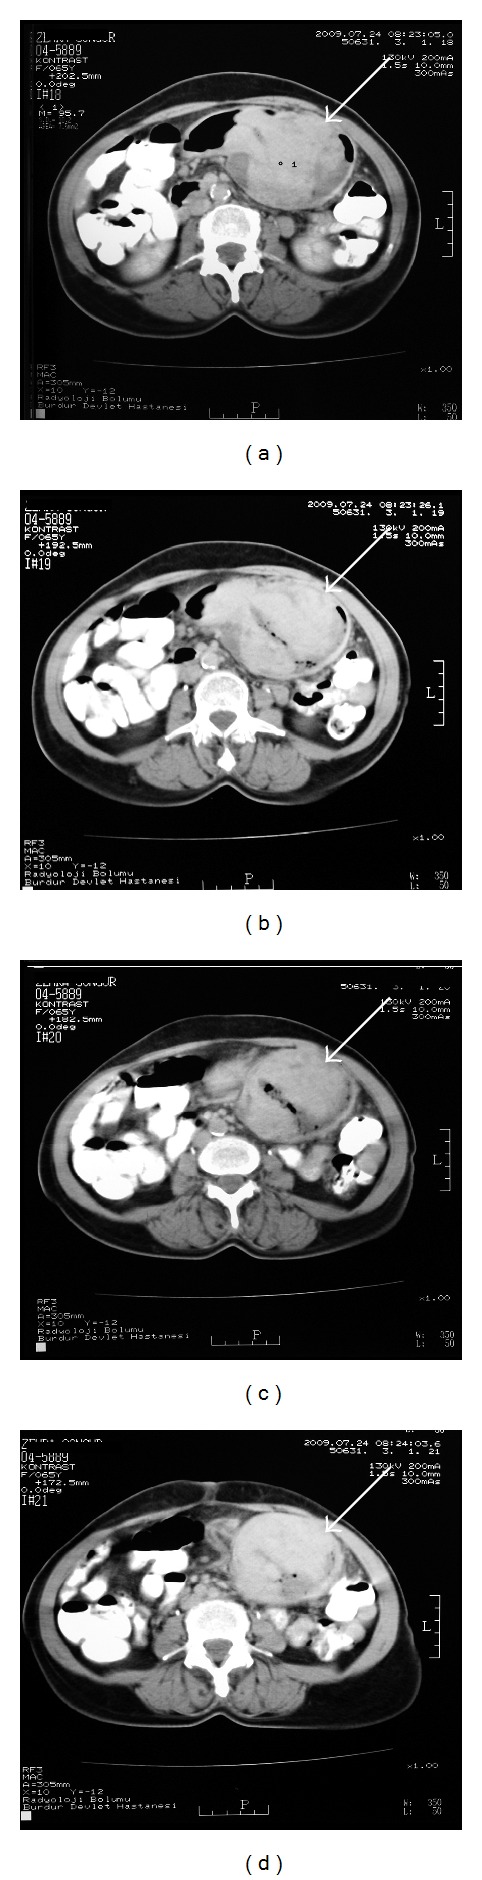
Abdomen computerized tomography (CT). In the right side of the abdomen midline, over the transverse column, a heterogeneous hyperdense solid mass with the size of 7,5 × 10 × 11 cm having cystic areas and air densities inside was determined (marked with an arrow).
